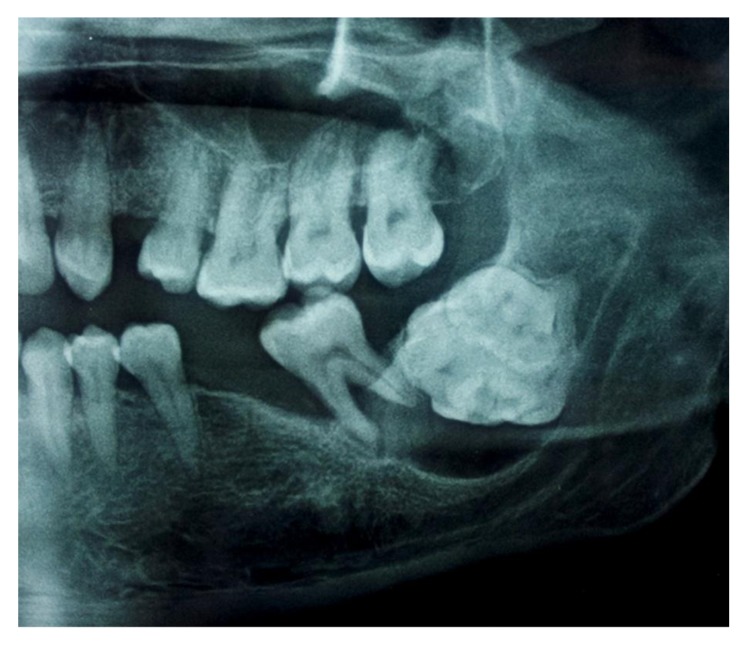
Panoramic radiograph revealing a well defined radiopacity in the left side of the mandible in the angle region with the secondary inferior displacement of the mandibular canal w.r.t 37.
